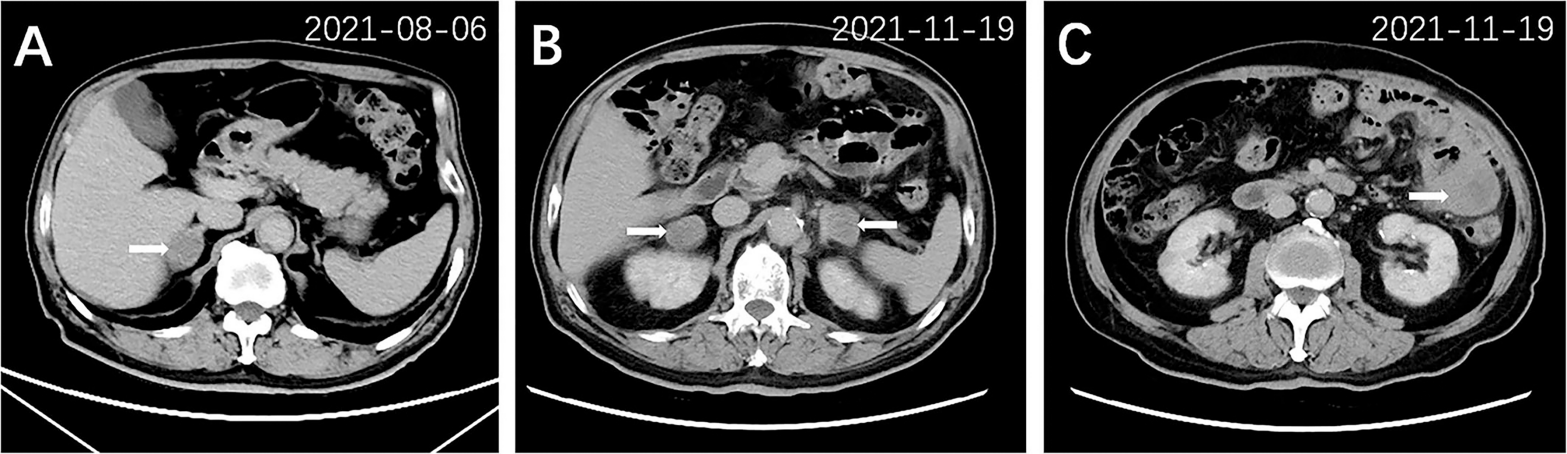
Abdominal CT scan of other metastatic lesions 3 months after treatment (A) and 6 months after treatment (B, C). A nodule (2.9 cm × 2.0 cm) in the right adrenal gland (arrow) was detected after 3 months of treatment (A). Multiple metastatic lesions were shown in bilateral adrenal glands (arrow, B) and left lower abdominal cavity (arrow, C) after 6 months of treatment.
